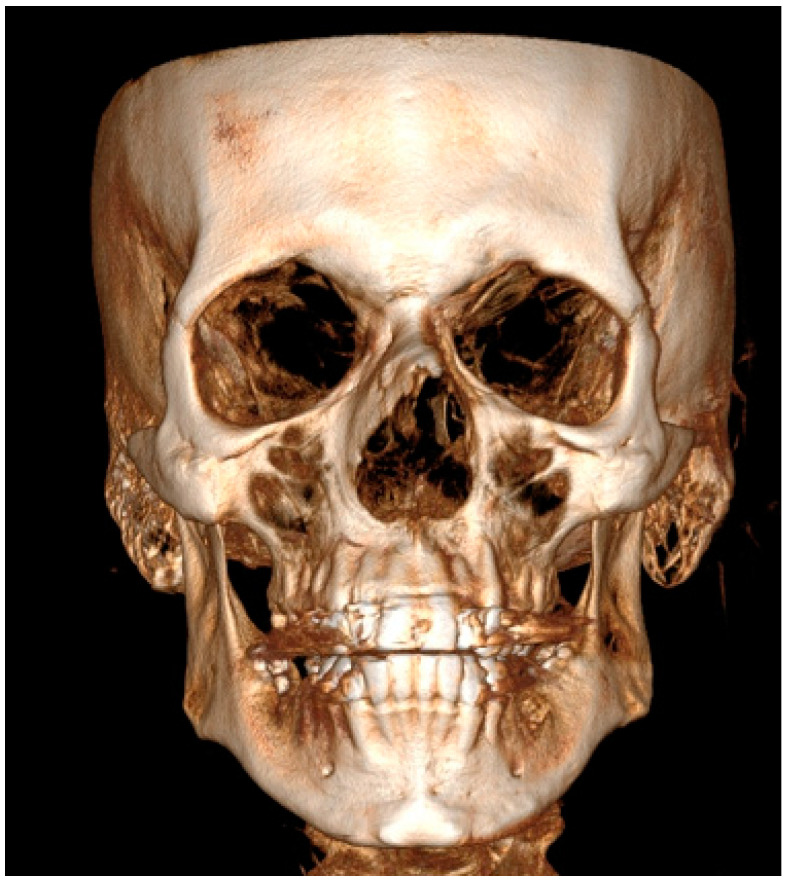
CBCT evaluation with 3D modeling in coronal view of a perfect symmetrical and balanced facial skeleton.
